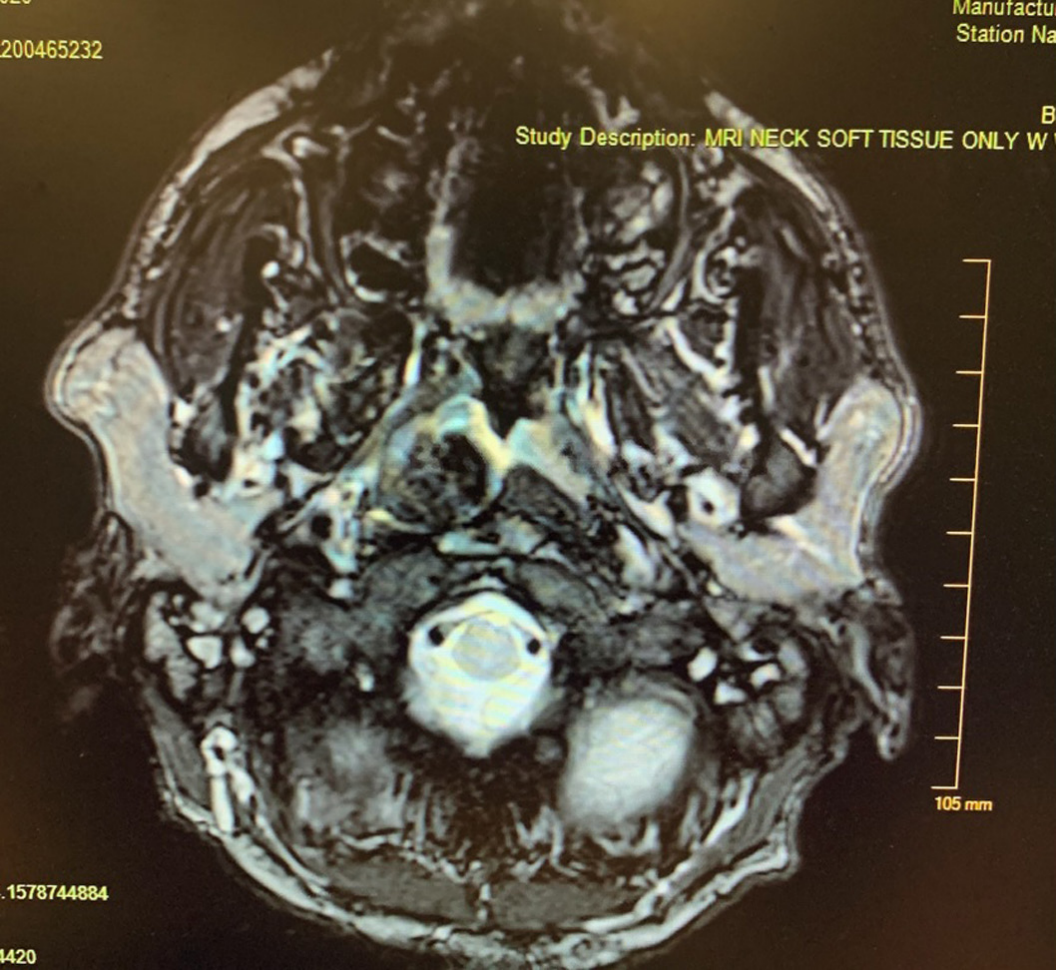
MRI soft tissue neck. Large, right‐sided parapharyngeal mass
